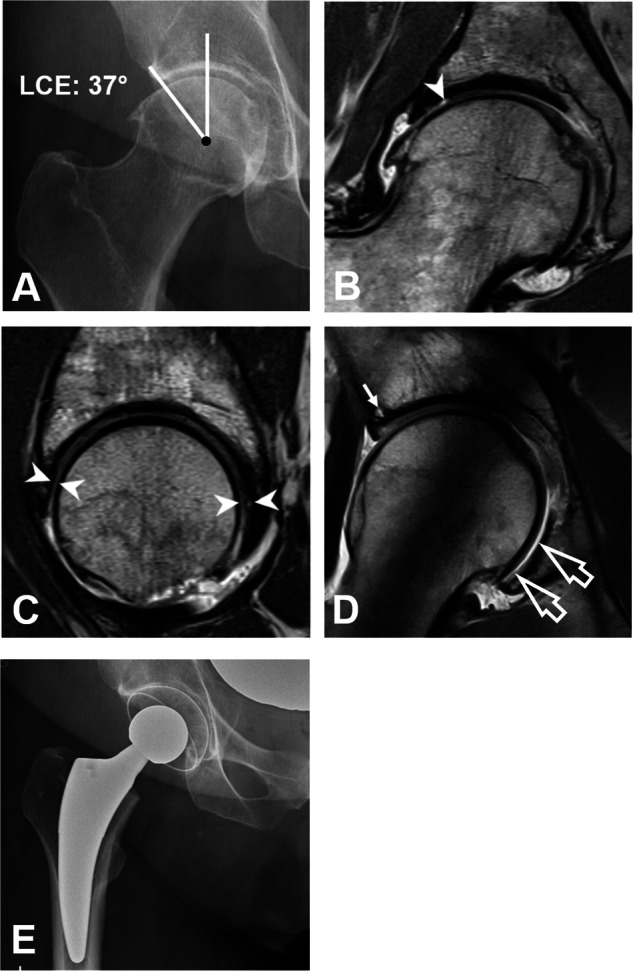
43-year-old man with hip pain due to mixed-type femoroacetabular impingement. A AP pelvis radiograph showing an increased lateral center-edge (LCE) angle of 37° and mild joint space narrowing. The patient underwent direct hip MR arthrography. B Coronal- and C sagittal- proton density-weighted turbo spin echo images showing cartilage damage involving more > 2 h on the clock-face (arrowheads). D Radial proton density-weighted turbo spin echo image demonstrates cartilage damage with a subchondral cyst (arrow). Femoral head decentration (open arrows) due to extensive joint damage is observed at the 7 o’clock position despite the presence of acetabular overcoverage. E Due to the advanced joint degeneration, the patient underwent subsequent total hip replacement
